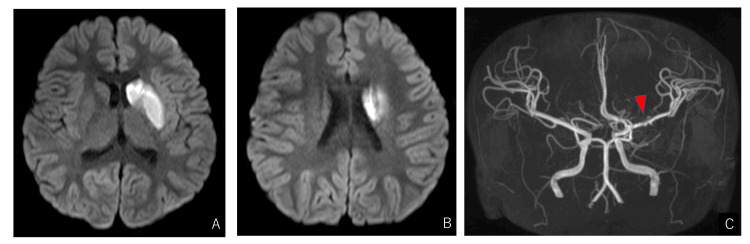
Magnetic resonance imaging at admission. Magnetic resonance (MR) imaging demonstrated diffusion restriction in the left basal ganglia (A, B). MR angiogram revealed irregular morphology in the horizontal portion of the left middle cerebral artery (arrow) (C).
